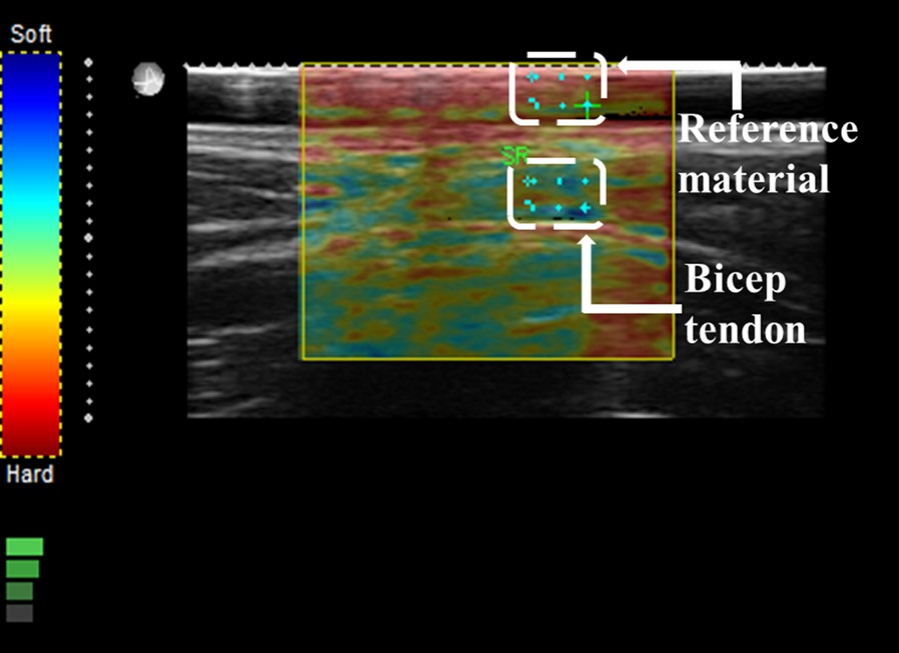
Ultrasonography measurement of distal bicep tendon muscle strain ratio using the reference material
